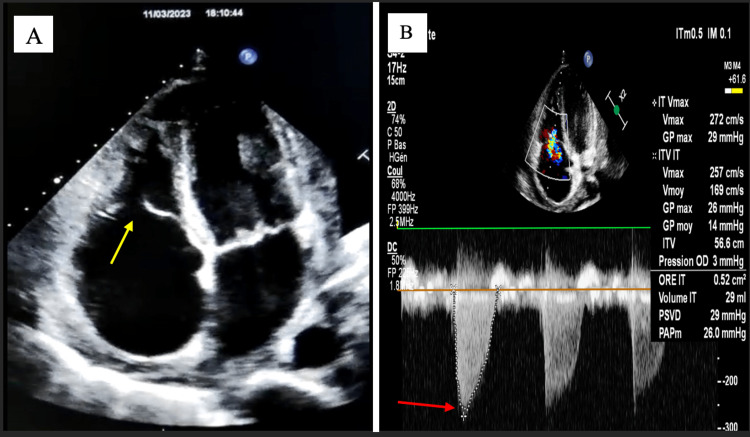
TTE findings one day after drainage A: Apical four-chamber view showing dilated right cavities with tricuspid valve diastasis (yellow arrow) B: Continuous wave doppler of the tricuspid regurgitation jet showing an envelope with triangular contour and early peak velocity (red arrow), which is indicative of severe tricuspid regurgitation
